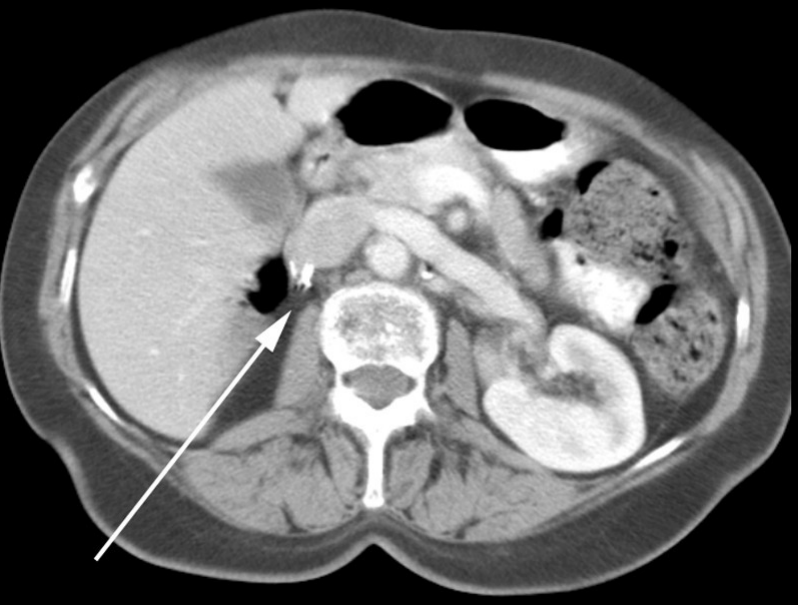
Computed tomography showing tumour mass in the renal bed (arrow points to surgical clips).
